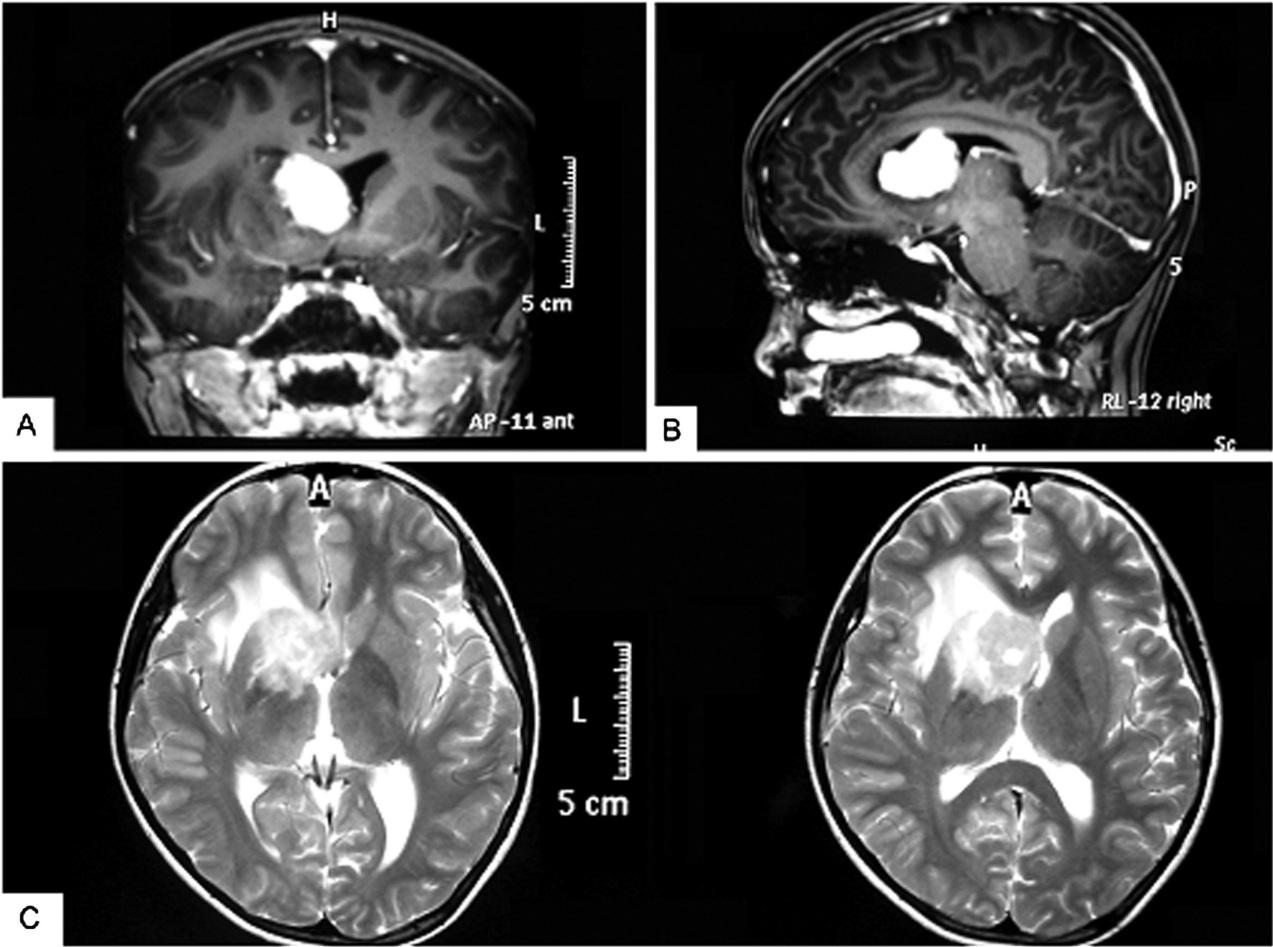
Brain magnetic resonance imaging of the patient before treatment. (A) A 3.4 × 2.3 × 2.4 cm mass in the anterior horn and body of the lateral ventricle with isointensity on the T1-weighted axial image. (B) Sagittal view of the same fragment. (C) Mass with slight edema on the T2-weighted image.
